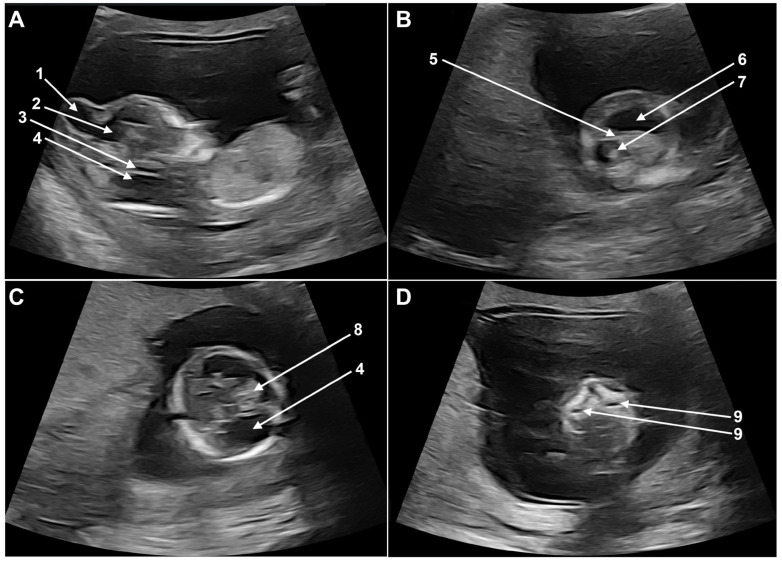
Fetal ultrasound images at the time of the first-trimester scan: (A) 1 + 2: cranial encephalocele and communication to right ventricle; 3: hypoplastic brain stem; 4: enlarged posterior fossa. (B) 5: asymmetric cerebral midline; 6: dilated left lateral ventricle; 7: right ventricle. (C) 8: choroid plexus; 4: enlarged posterior fossa. (D) 9: prominent maxillary cavities.
